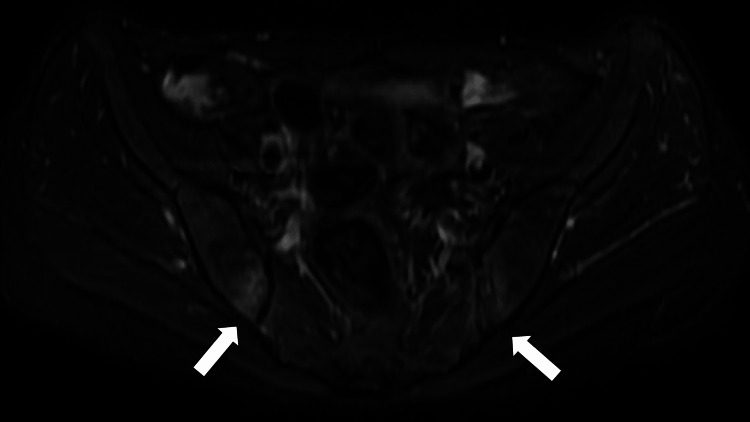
Pelvic magnetic resonance imaging (short inversion time inversion recovery) showing a high-intensity lesion on the joints, indicating the presence of sacroiliac joints inflammation (white arrows)
